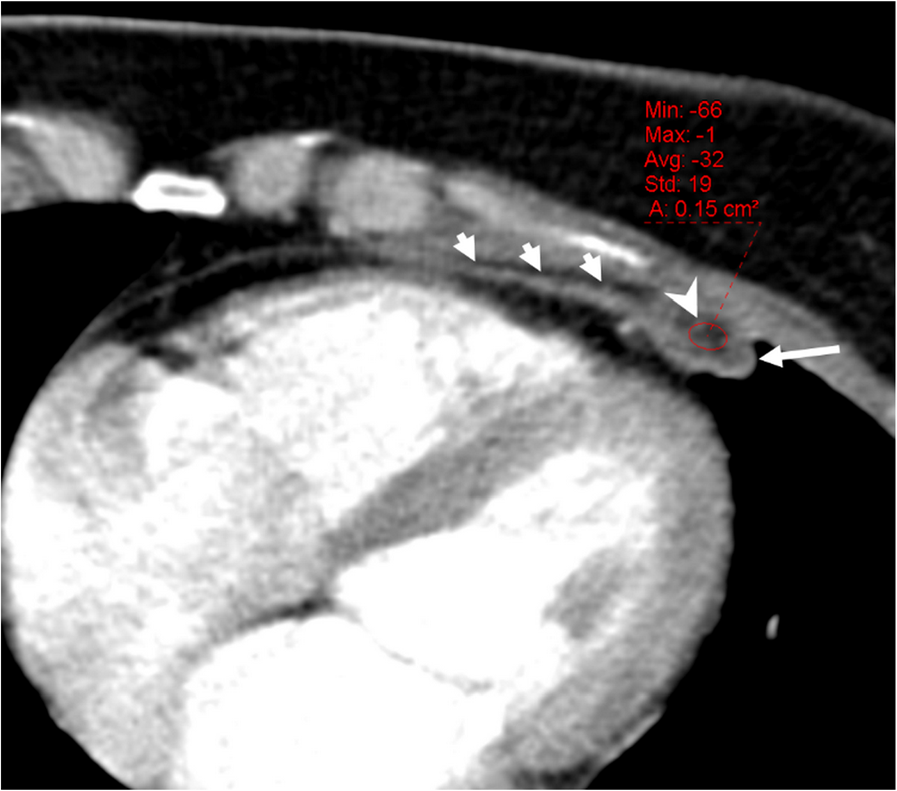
A 46-year-old woman with a 5-day history of chest pain and shortness of breath. Axial contrast-enhanced CT image demonstrates the fat-containing (arrowhead) soft tissue thickness (long arrow) and associated pericardial thickening (short arrows) compatible with pericardial/epipericardial fat necrosis. The CT findings resolved after conservative treatment
